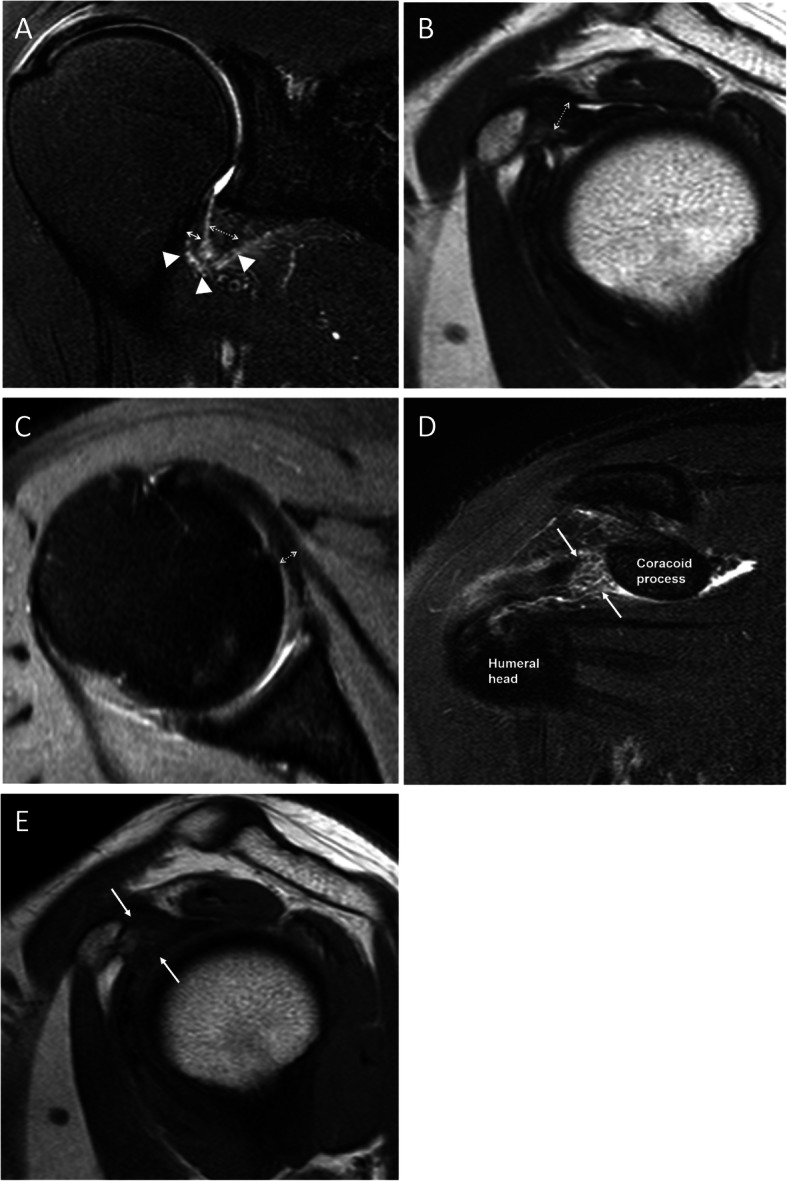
Examples of findings on magnetic resonance images for a 48-year-old woman with adhesive capsulitis. a. Oblique coronal fat-suppressed T2-weighted image showing measurement of the thickest portion of the axillary joint capsule in both humeral (arrow) and glenoid (dashed arrow) attachment and also showing axillary capsular thickening and abnormal hyperintensity (arrow heads). Increased thickness was present only at the glenoid portion (6.23 mm); thickness was normal at the humeral portion (2.81 mm). b Oblique sagittal T2-weighted image showing measurement of the coracohumeral ligament thickness (dashed arrow). c Axial fat-suppressed proton density image showing measurement of anterior capsular thickness (dashed arrow) below the subscapularis tendon. d Oblique coronal fat-suppressed T2-weighted image at the coracoid process level showing abnormal hyperintensity in the subcoracoid fat triangle (arrows). e Oblique sagittal T1-weighted image showing obliteration of the subcoracoid fat triangle (arrows)
